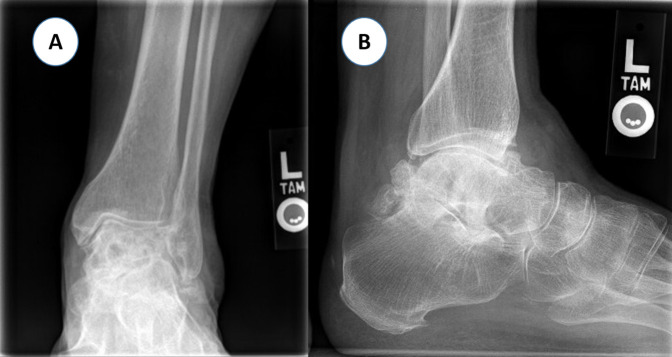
Anteroposterior (A) and lateral (B) plain radiograph of the left ankle demonstrating extensive mixed area of sclerosis and luscencies within the talus with flattened talar dome, partial talar collapse, and secondary degenerative osteoarthritis of the tibiotalar and subtalar joints.
